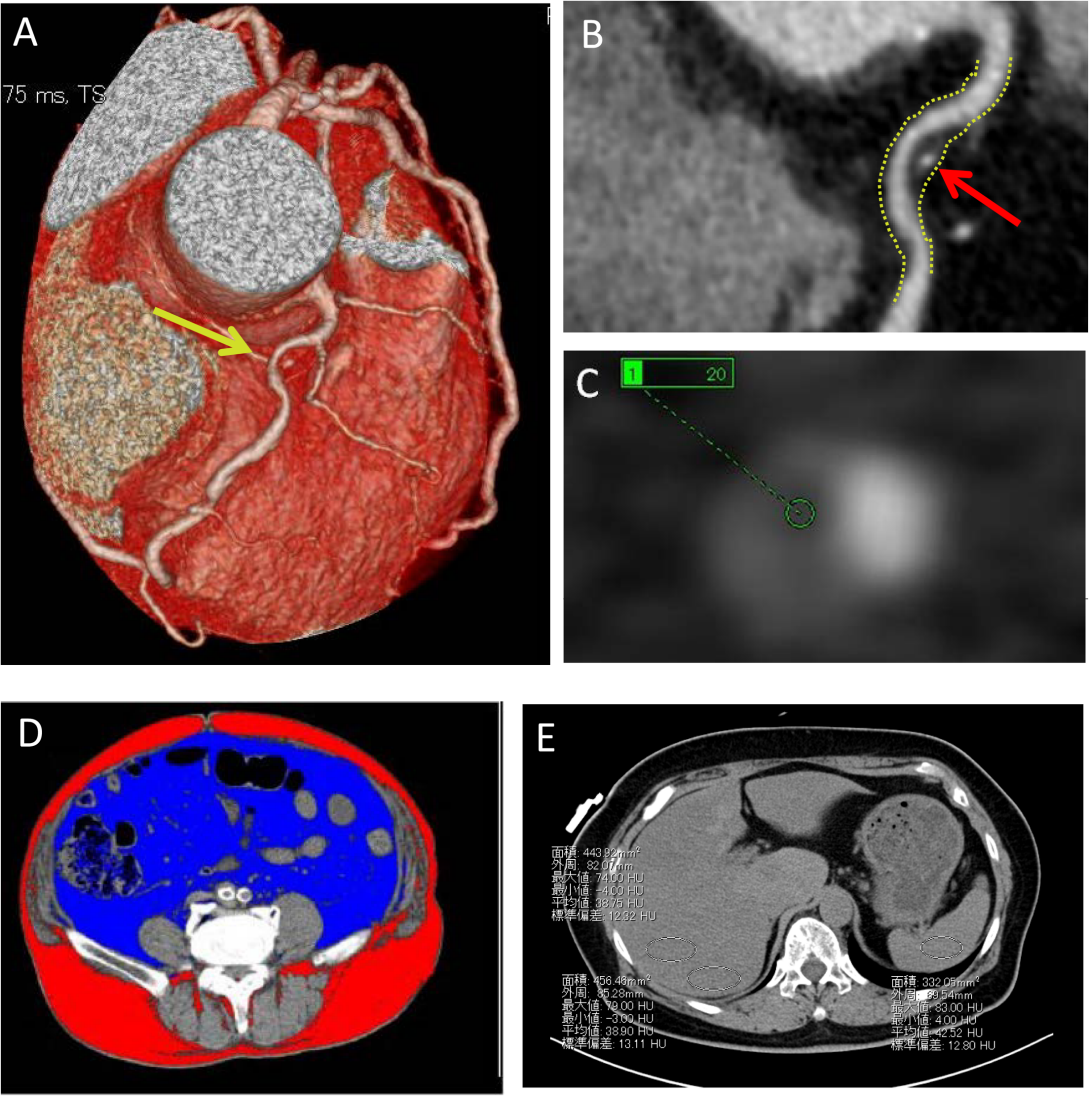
CT images of a coronary plaque, visceral adipose tissue, and liver. (A), Multidetector computed tomography imaging of a high-risk plaque with volume rendering; (B), curved multi-planar reconstruction image; and (C), an axial image. The yellow arrow in (A) denotes a high-risk plaque site. This lesion was positively remodeled as shown by comparison with a normal coronary segment proximal to the lesion (yellow dotted line in (B)). The red arrow in (B) denotes a region of spotty calcification, which is a low-density plaque of 20 HU. (D) CT of the liver of a patient with nonalcoholic hepatic steatosis. (E) CT image of a high area of visceral adipose tissue (220.4 cm^2^). Diffuse fat accumulation is observed in the liver in an unenhanced CT image with an L/S ratio of 0.74.
